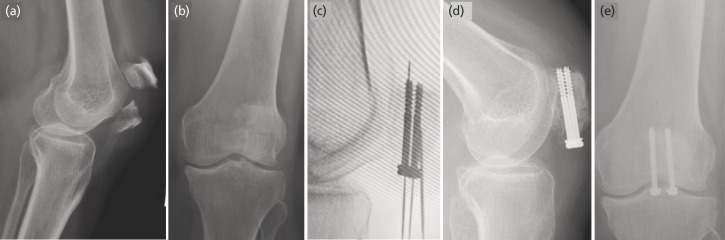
(a) and (b) plain radiograph show transverse patellar fracture (Classification; AO 34C1). (c) Cannulated screw fixation inserted with the guidance of guide wire and image intensifier. (d) and (e) radiographic union over fracture were evaluated during follow-up.
